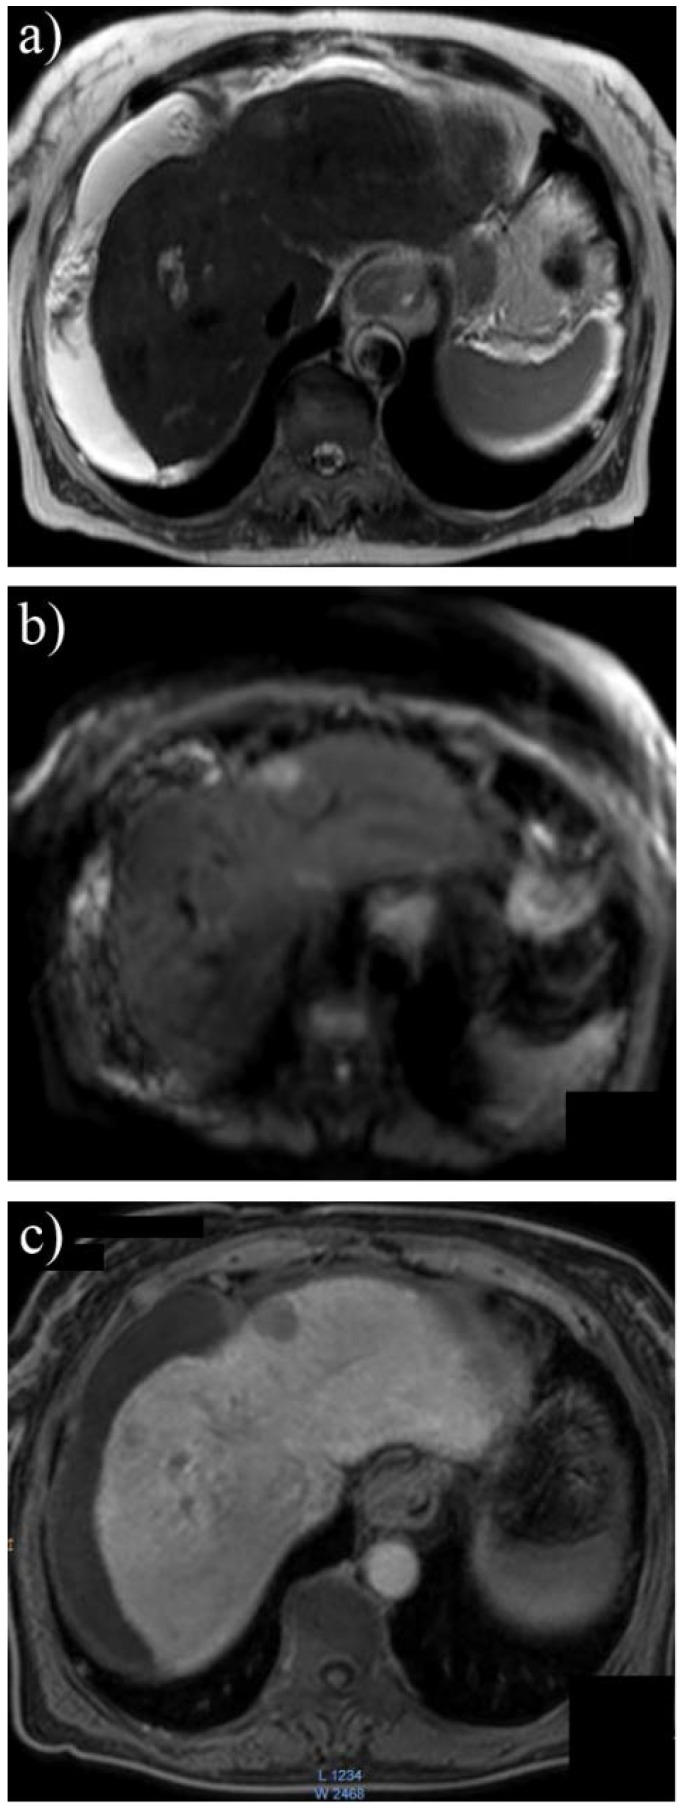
3T MRI in a patient with small HCC in segment II with high signal intensity in T2 weighted (a) and diffusion (b) sequences and decreased signal intensity at 30 minutes after contrast enhancement with hepatospecific paramagnetic gadolinium based contrast agent (c)
